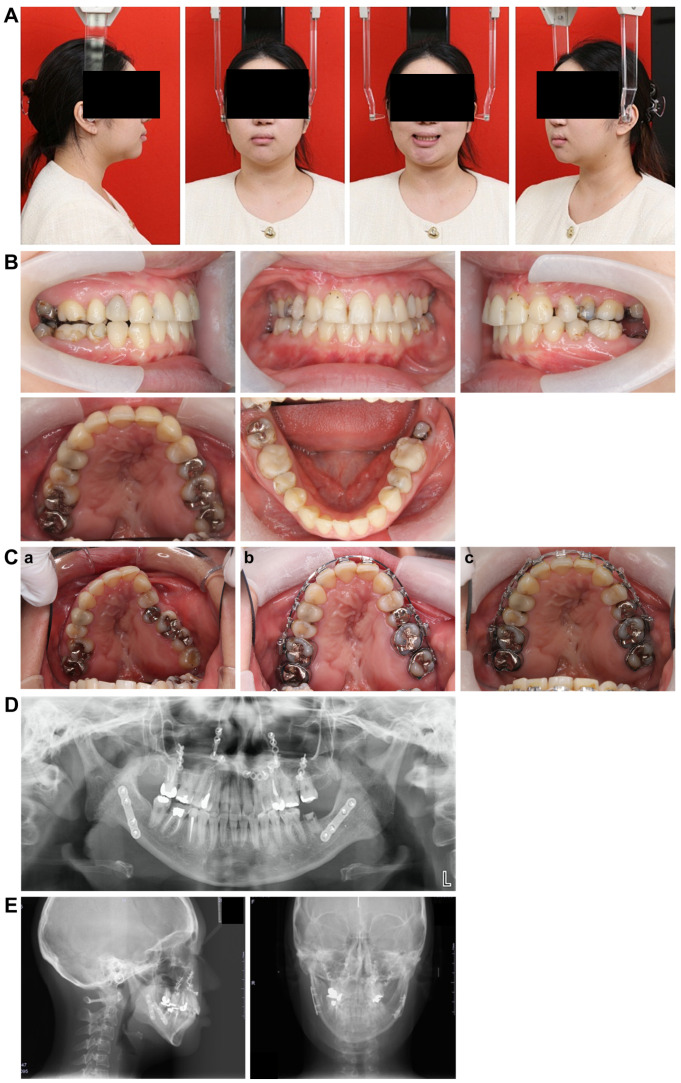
Post-treatment record. (A) Facial photographs. (B) Intraoral photographs. (C) a: at the time of initial examination: anterior width 25.1 mm, posterior width 38.0 mm; b: at the end of preoperative correction: anterior width 29.1 mm, posterior width 38.7 mm; c: immediately before retention: anterior width 34.2 mm, posterior width 43.8 mm. (D) Panoramic radiograph. (E) Lateral and front cephalogram.
